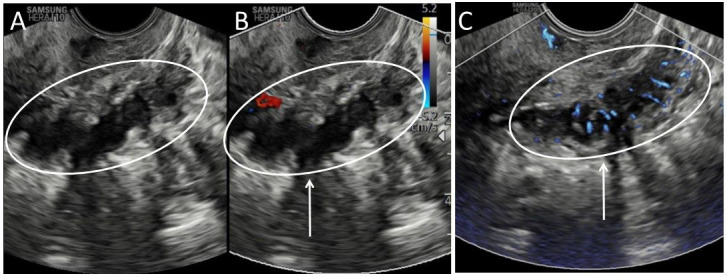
Representative image of a deep infiltrating endometriosis lesion. A. Endometriosis in B-mode; B. Endometriosis in Directional power Doppler mode; C. Endometriosis in MV-flow^TM^ mode. Arrows indicate deep endometriosis lesion with infiltrating vessels, only visible in MV-flow^TM^ mode.
